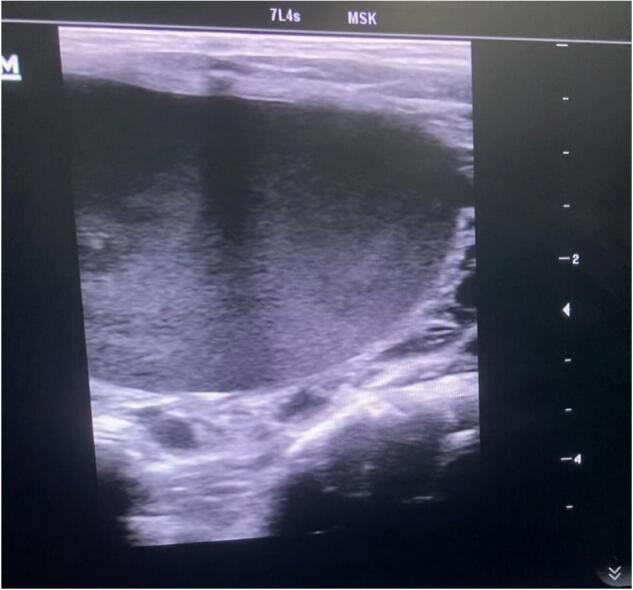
An ultrasound of the neck demonstrated lesion measures about (3.5 × 5 × 6 cm), located at the base of the neck without solid components, which suggests cervical cystic hygroma.
